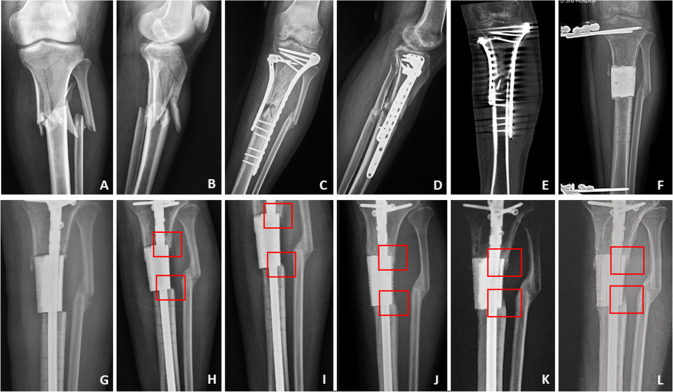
The therapeutic process of the second typical case. A, B The initial X-ray displayed the patient suffered the tibial comminuted fracture; C–E the patient underwent the surgical treatment, and at 10 months after the initial surgery, X-ray and CT scan showed fracture nonunion and sequestrums; F we cleared the sequestrums and filled a PMMA spacer into the femoral defect with 6.3 cm length, and stabilized the femur by external fixator; G–L after an 8-week interval, the bone defect was repaired by a customized 3D printed scaffold, and the postoperative regular X-rays also showed the scaffold kept stable and new bone grew gradually on the surface of scaffold from both ends of the defect (as displayed in the red boxes)
